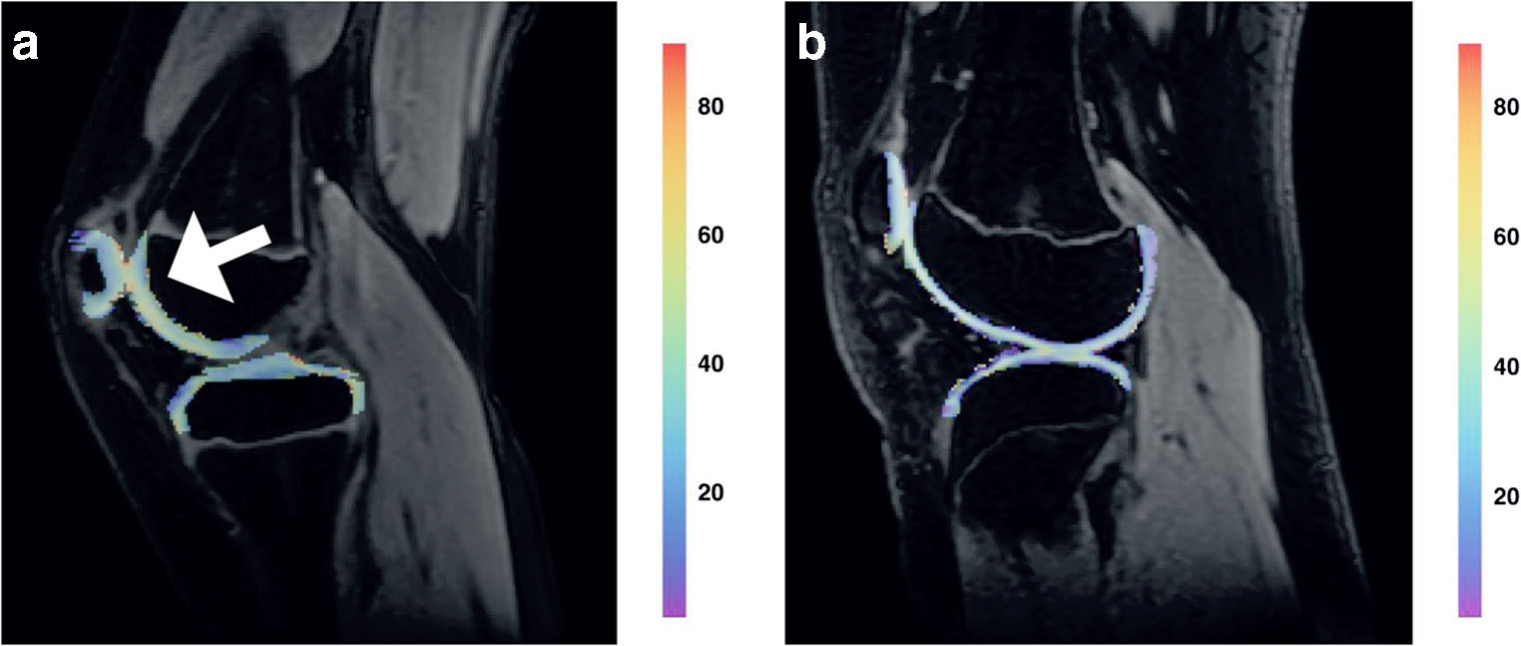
Two T_1ρ_ MR imaging examples in children from this study. a, b T_1ρ_ overlay image (a) of a 10-year-old boy with oligo-articular juvenile idiopathic arthritis who had a swollen and warm right knee with slight limitation of motion on examination. On the image, higher T_1ρ_ values are observed as compared to the child depicted in (b). Especially the cartilage in the patellofemoral joint demonstrates high T_1ρ_ values (*arrow*). In this boy, synovial inflammation was observed on MR imaging in the patellofemoral synovium and around the cruciate ligaments. T_1ρ_ overlay image (b) of a 13-year-old girl who presented with knee pain. She was suspected of having juvenile idiopathic arthritis. On examination, minor swelling of the knee was found. The conventional MRI showed no synovial inflammation in the knee. T_1ρ_ values are markedly lower as compared to (a). Note the higher relative contribution of purple and blue in the cartilage overlay. After clinical, laboratory and imaging evaluation she was diagnosed with a functional pain syndrome
